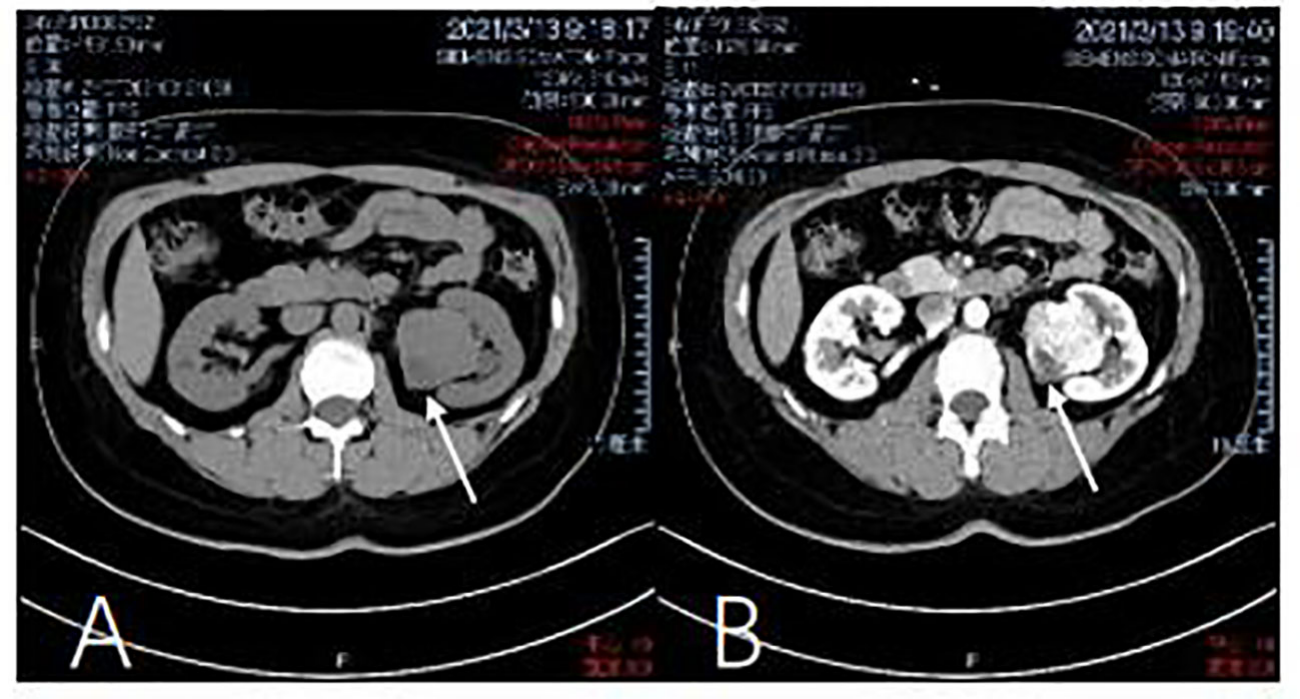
Computed tomography of the kidney. (A) Abdominal pelvis CT scan shows a mass in the left kidney. There is a no dular mass of about 4 x 3.3 x 3 cm in the middle and lower pole of the kidney. (March 13, 2021). (B) Contrast-enhanced CT shows heterogeneous enhancement in the cortical phase (March) 13, 2021).
